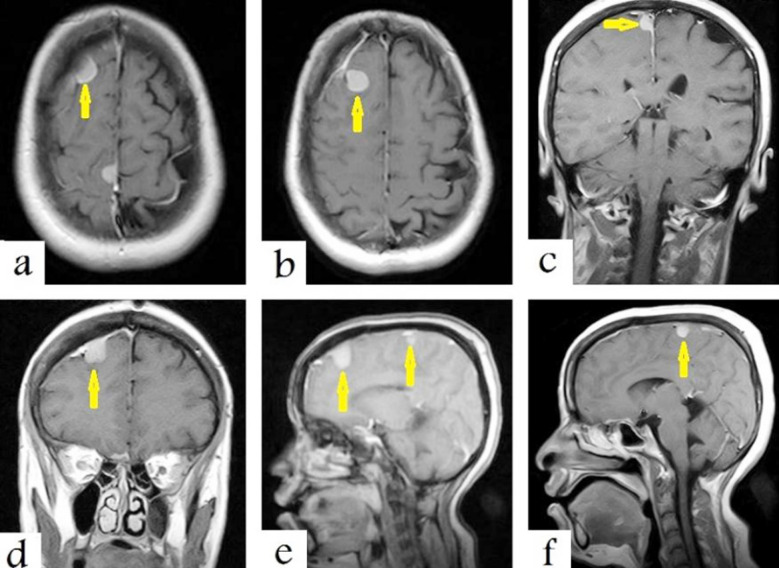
Selected T1-weighted MRI images with contrast administration show other brain tumours with avid enhancement on axial (a and b), coronal (c and d), and sagittal (e and f) sections, which are typical in images of convexity and parasagittal meningiomas (arrows).
